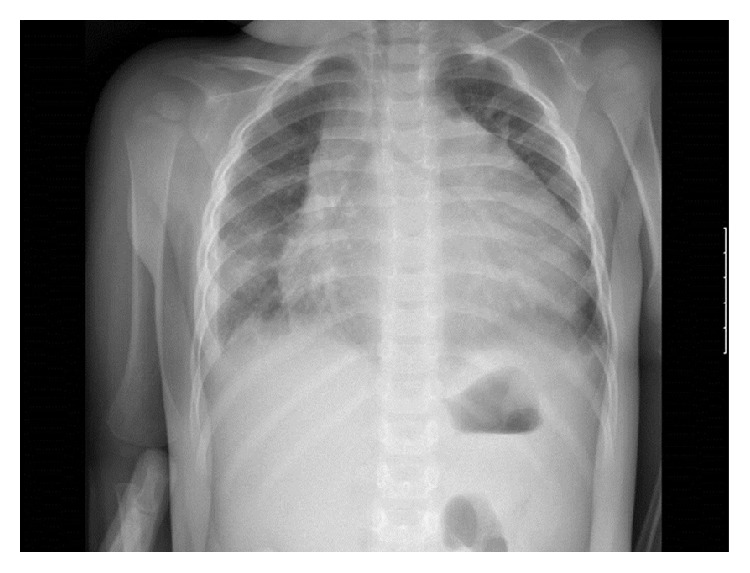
Chest X-ray. Increase of the cardiothoracic index at the time of diagnosis of cardiac tamponade. The patient had a normal chest X-ray 10 days before.
